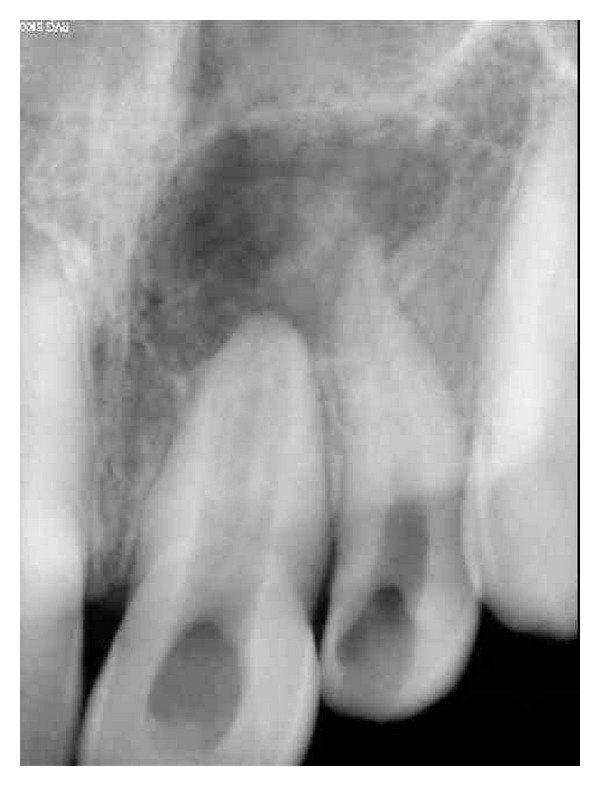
Radiograph revealing the removal of broken bur, also note the coronal flaring that allowed the easy removal of the bur fragment.
